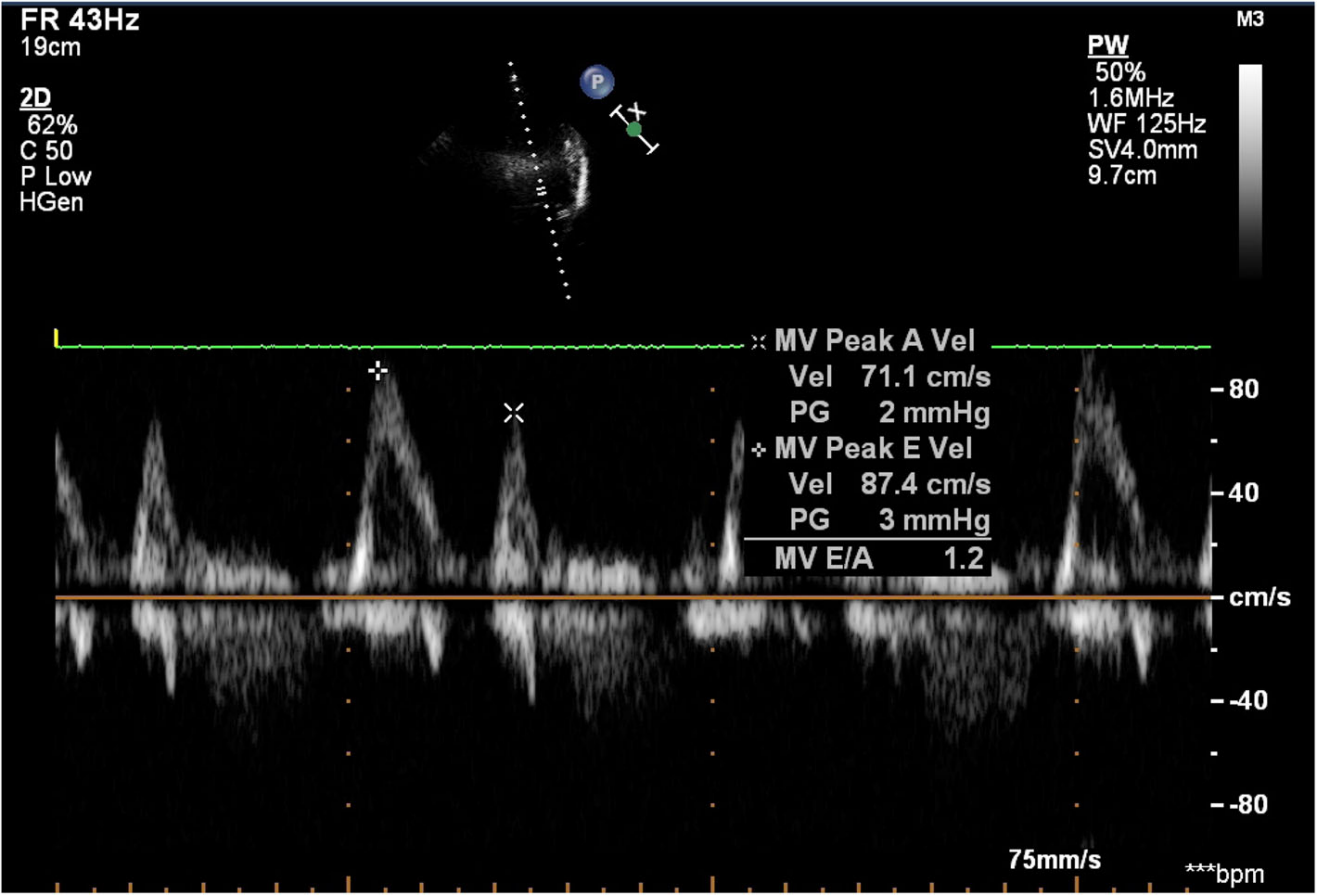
Assessment of diastolic function by transmitral inflow pulsed wave Doppler study
